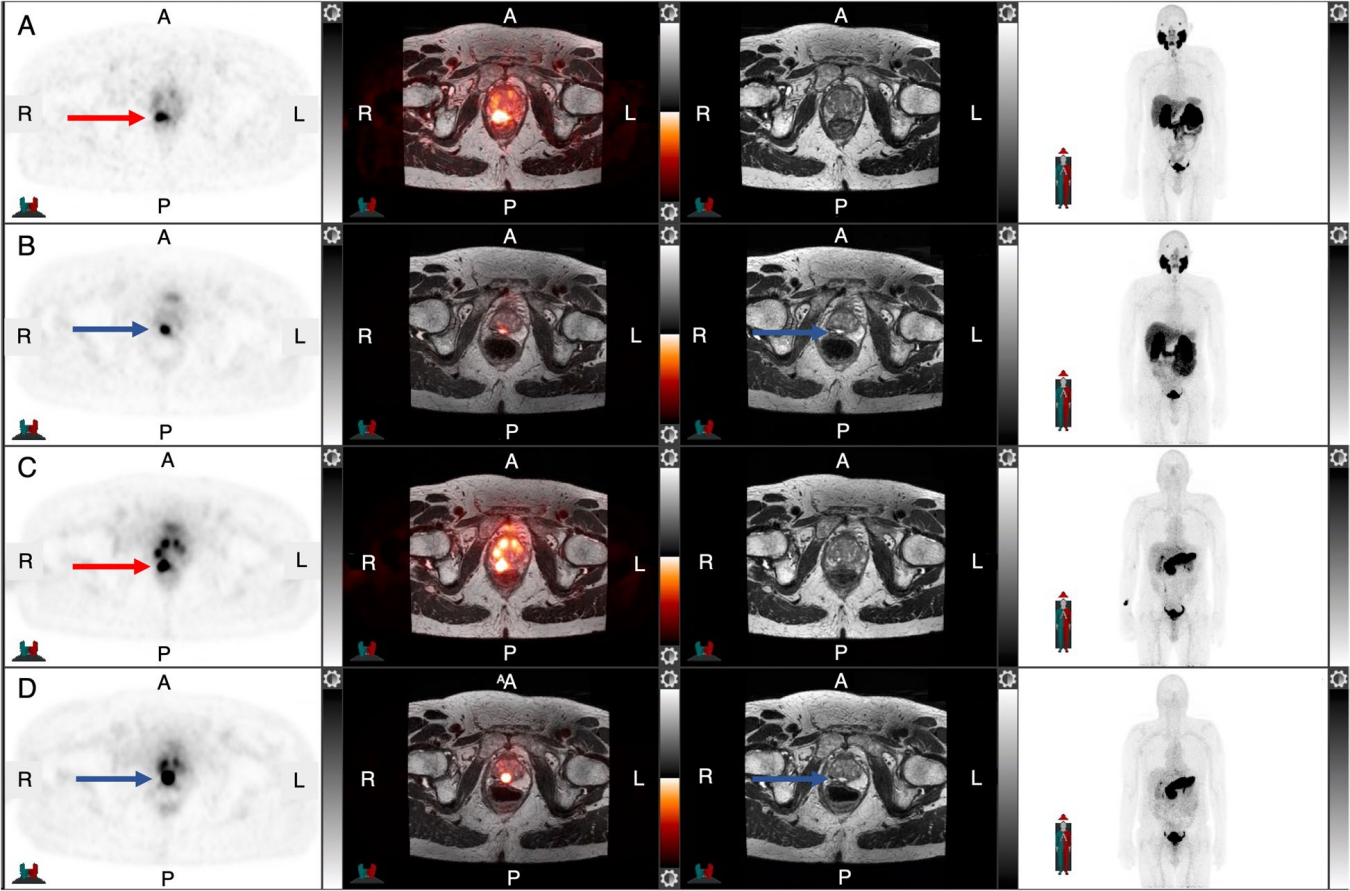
78-year-old man presents with PSA 15.90 ng/mL and PSA density 0.14 ng/mL^2^ for PET-targeted HIFU of a PI-RADS 5 lesion in the right posterior apex. Pre-therapy ^68^ Ga-PSMA11 A and ^68^ Ga-RM2 C axial PET, axial fused PET/MRI, MRI, and MIP images show concordant focal uptake in the right posterior prostate (red arrows). After HIFU, ^68^ Ga-PSMA11 B and ^68^ Ga-RM2 D axial PET, axial fused PET/MRI, MRI, and MIP show structural changes with urine pooling in the treated area (blue arrows) but no pathologic uptake. Biopsy confirmed no evidence of PC in the treated area
